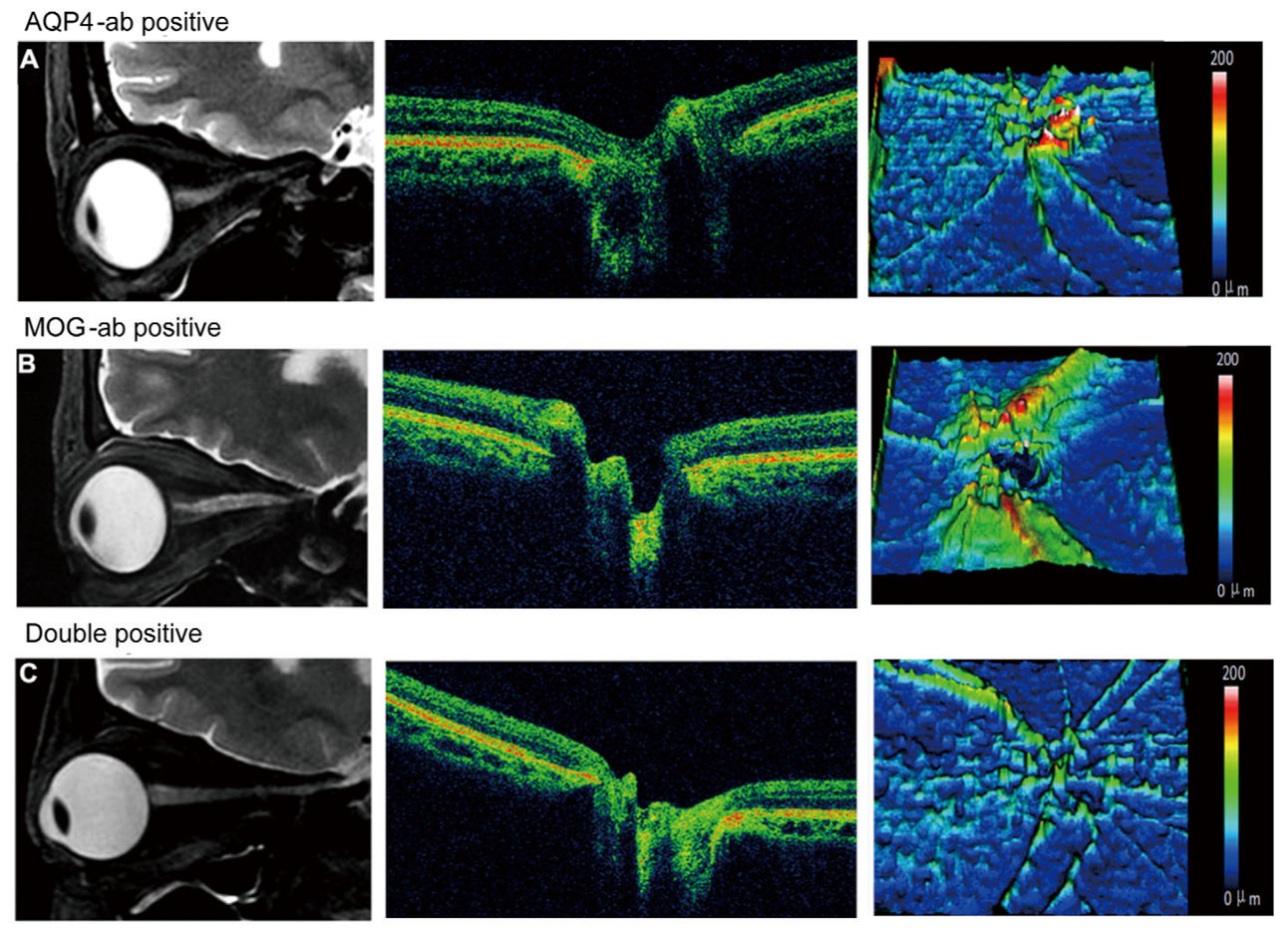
Optica nerve and retinal nerve fiber layer thickness in NMOSD patients with different antibody profiles at the last follow-up. A, Images from a patient with AQP4-ab and a 5-year course of disease. B, Images from a patient with MOG-ab and a 5-year course of disease. C, Images from a patient with both AQP4-ab and MOG-ab and a 2-year course of disease. Left, optic nerve MRI. Middle, Optical coherence tomography images of the peri-papillary area of retinal nerve fiber layer. Right, Thickness maps of the retinal ganglion cell layer of retinal nerve fiber. Optica nerve demyelination is evident as atrophy on optic nerve MRI, and neuroaxonal retinal damage appears as thinning of peri-papillary tissue measured by OCT. This condition was more severe in eyes of patients with optic neuritis who had AQP4-ab and MOG-ab than in those with either AQP4-ab alone or MOG-ab alone.
